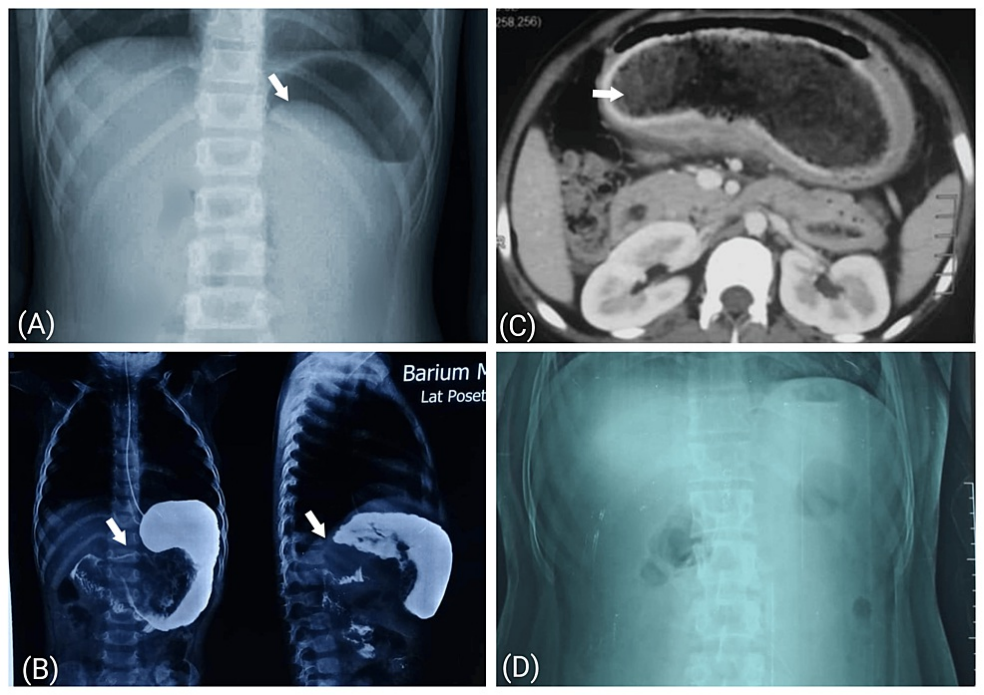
Erect abdomen X-ray showing ambiguous shadow (A) in the epigastrium and left hypochondrium (white arrow), barium meal (B); showing a filling defect in the gastric region, CT-scan (C); showing typical mottled appearance, and X-ray erect abdomen showing no significant findings (D) in a patient diagnosed with persimmon-related phytobezoar before (A)-(C) and after (D) intervention. CT: computed tomography.
